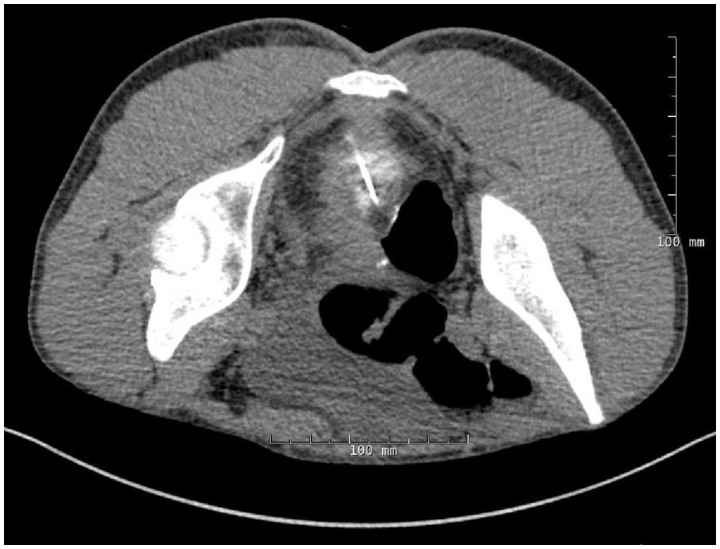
CT image showing thin guide-wire placement (Accura^™^ II breast localization needle) from buttock to posterior wall of the mid pouch.
